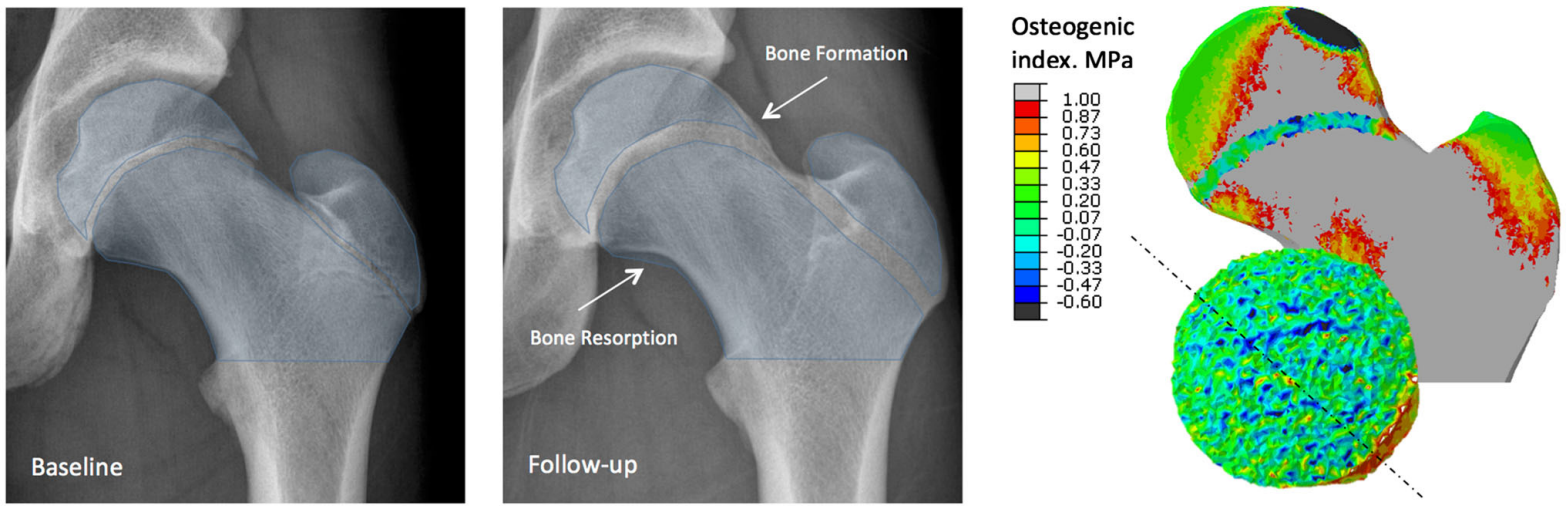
An X-ray of a representative young soccer player at baseline and follow-up, together with the distribution of the osteogenic index close to the growth plate. As the femur grows, there are areas of bone formation, and possibly even areas of bone resorption. These observations nicely explain load-driven development of a cam-type deformity. Reprinted from Roels et al. [17], with permission from the Osteoarthritis Research Society International. © 2014
